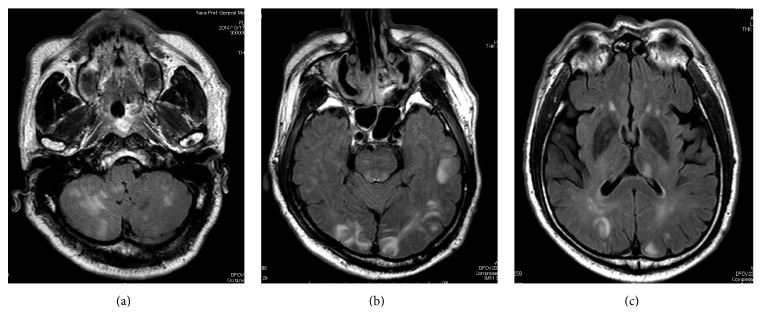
MRI (FLAIR images) of the brain on admission. Multiple high-intensity lesions in the white matter of the occipital and cerebellar lobes, dorsal brain stem, and left thalamus were demonstrated, suggesting RPLS. ((a) Cerebellar lobes lesions, (b) occipital lobes and dorsal brain stem lesions, and (c) left thalamus and occipital lobes lesions.)
